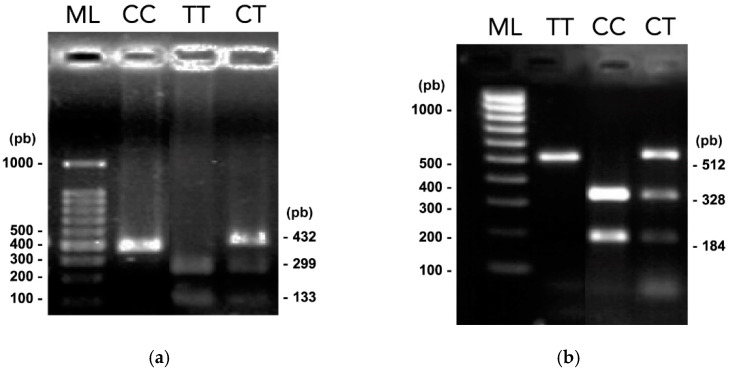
PCR-RFLPs results. Electrophoresis in 1.5% agarose gels of digested amplified products with *Bpu10I* and *HpyCH4IV* restriction enzymes (related to Figure S1) (a) Digested amplified products of SNP *TOX3* rs3803662. (b) Digested amplified products of SNP *MMP7* rs1943779. TT, CC, and CT genotypes are shown, and lane ML indicates the 100 to 1000 bp molecular gene ruler ladder.
